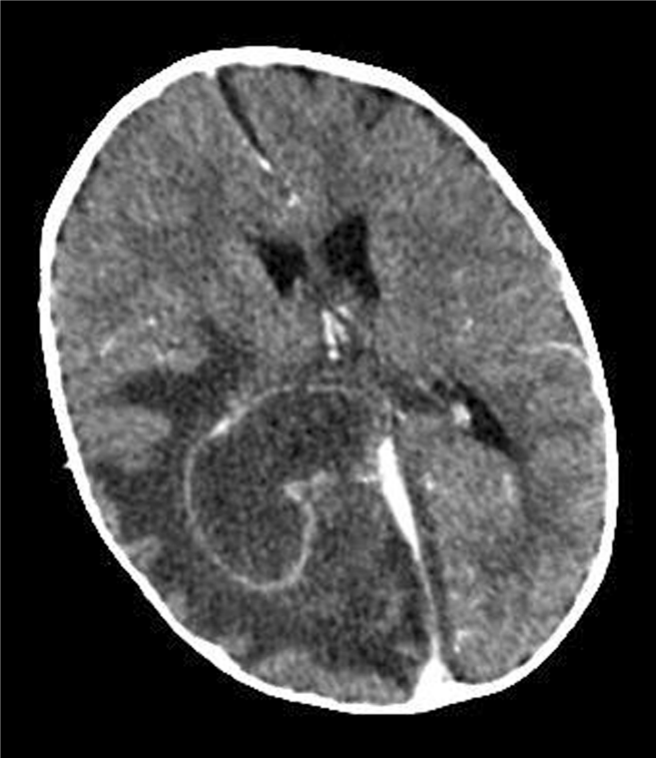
Pre-operative contrast enhanced CT scan.
